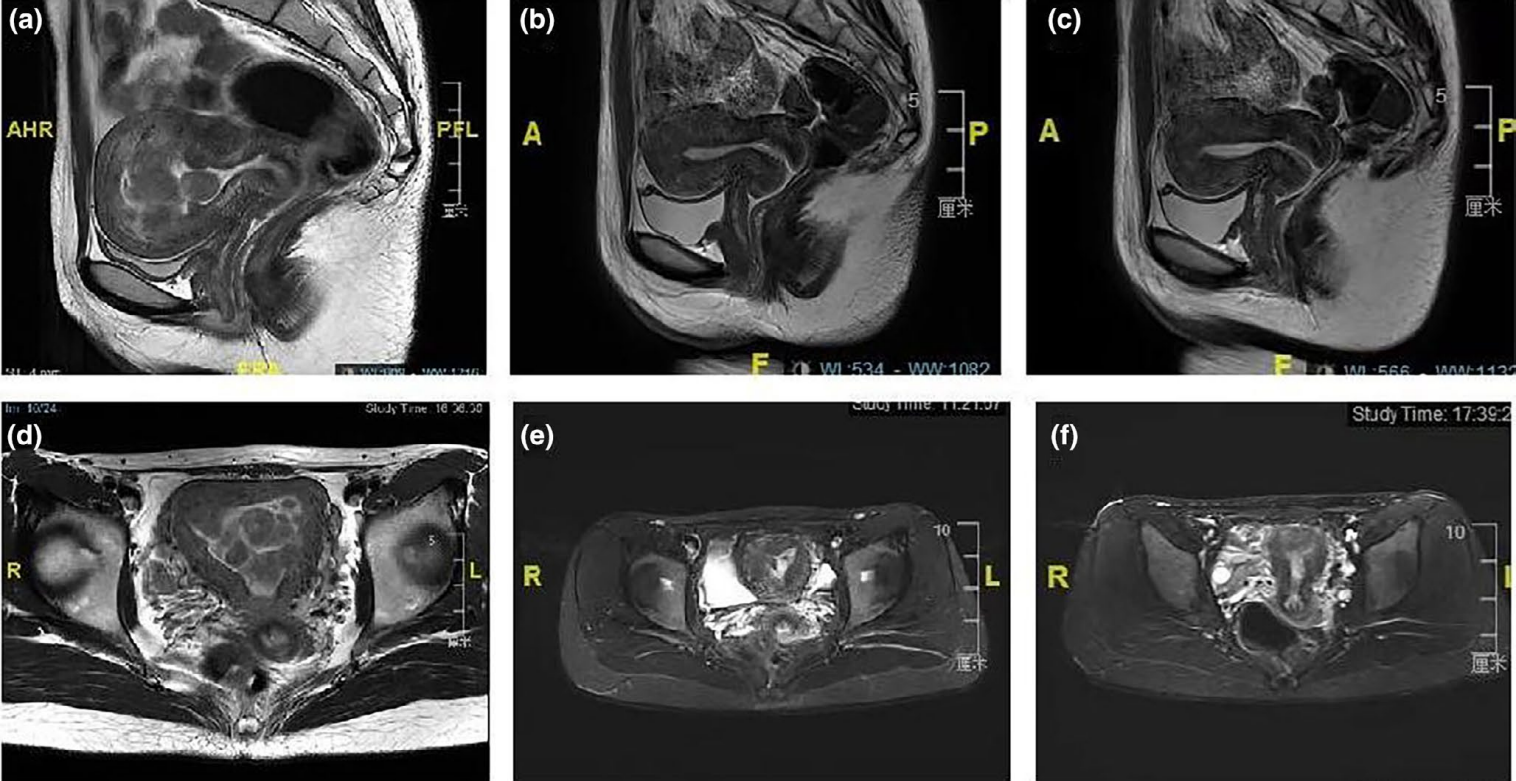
Pre‐ and postoperative pelvic magnetic resonance imaging (MRI) T2‐weighted images. (a) Preoperative sagittal view showing diffusely distributed submucosal leiomyomas in the uterine cavity. (b) One‐month postoperative sagittal view. (c) One‐year postoperative sagittal view. (d) Preoperative coronal view. (e) One‐month postoperative coronal view. (f) One‐year postoperative coronal view.
